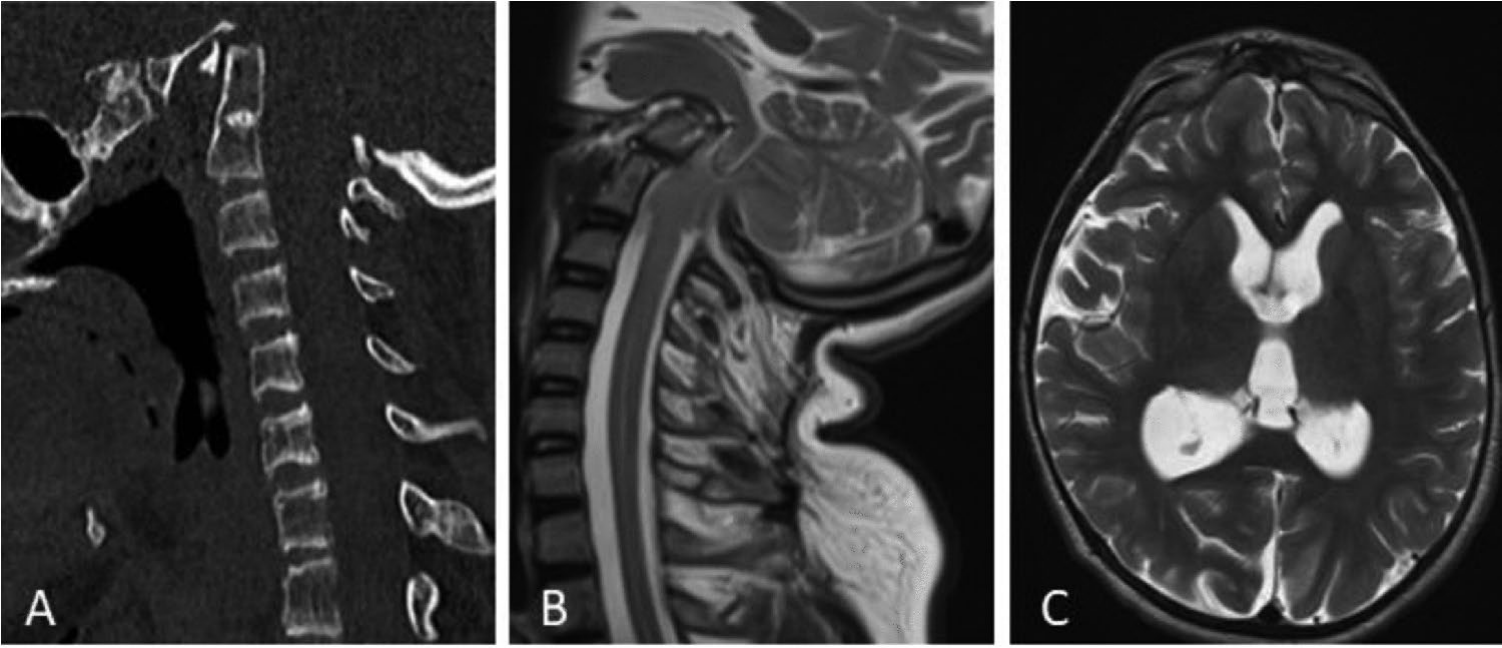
Preoperative imaging: A Sagittal CT scan showing basilar impression. B Sagittal T2-weighted MRI showing brainstem compression due to prolapse of the dens in the foramen magnum. C Axial T2-weighted MRI showing hydrocephalus due to CSF impaired circulation at the CVJ
